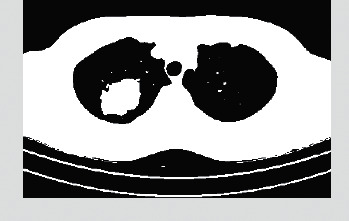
CT image after preprocessing.
